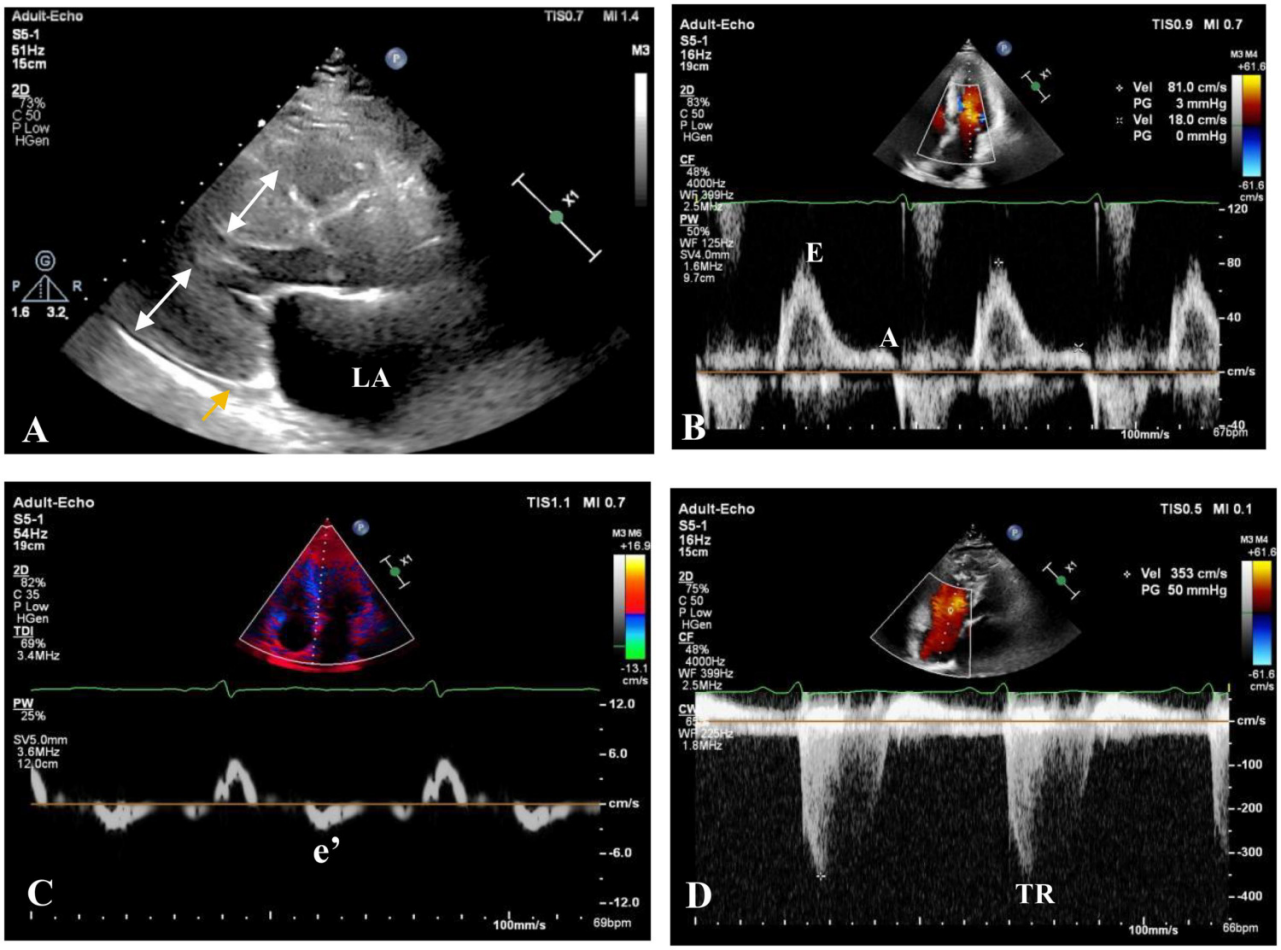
Transthoracic echocardiogram showing typical echocardiographic features of cardiac amyloidosis: (A) parasternal long-axis view showing severe concentric left ventricular hypertrophy (white arrow), sparkling granular appearance of the myocardium, minimal pericardial effusion (yellow arrow), and left atrial(LA) enlargement. (B) Transmitral valve inflow diastolic peak early (E 0.82 m/s)/later (A 0.18 m/s) velocity obviously increased. (C) Tissue Doppler imaging showing markedly reduced septal early diastolic velocity of the mitral valve annular (e′ 3 cm/s). (D) Elevated tricuspid regurgitation velocity (TR 3.53 m/s) consistent with pulmonary hypertension.
